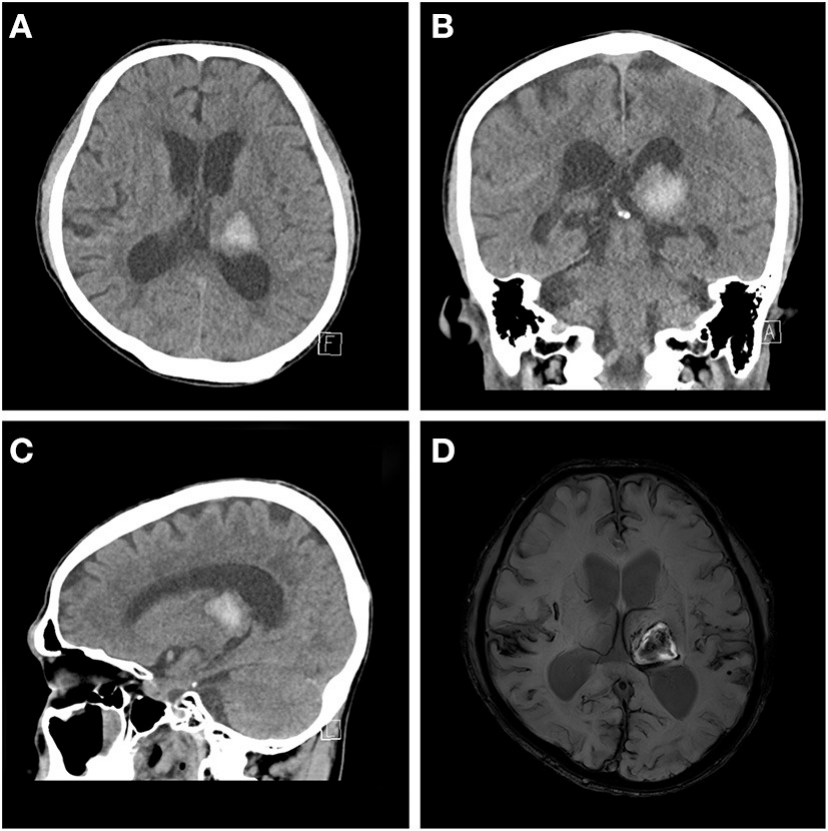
Baseline brain magnetic resonance imaging and computed tomography (CT) scan. Acute intracerebral hemorrhage at the left thalamus is observed on axial (A), coronal (B), and sagittal views (C) of the brain CT scan and the axial view of the diffusion-weighted image (D).
